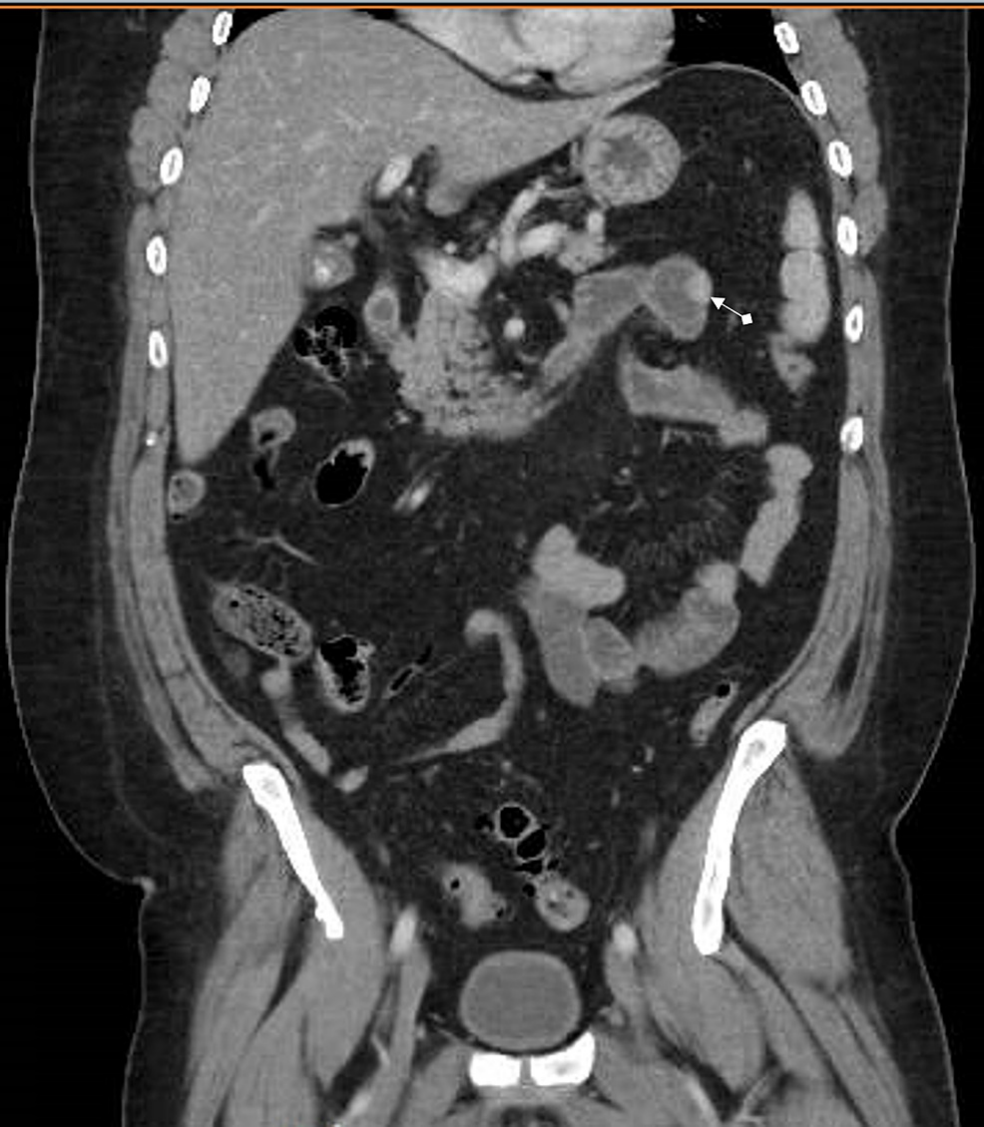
Coronal section of CT abdomen with IV contrast showing 1.5 cm hyperdense, circular jejunal lesion with regular borders in the left upper quadrant.
